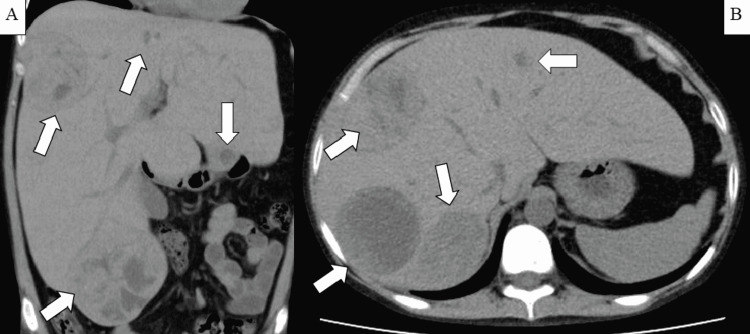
Chest-abdominal non-contrast computed tomography (A, coronal plane; B, transverse plane) The image shows enlarged liver with multiple adenomas.
